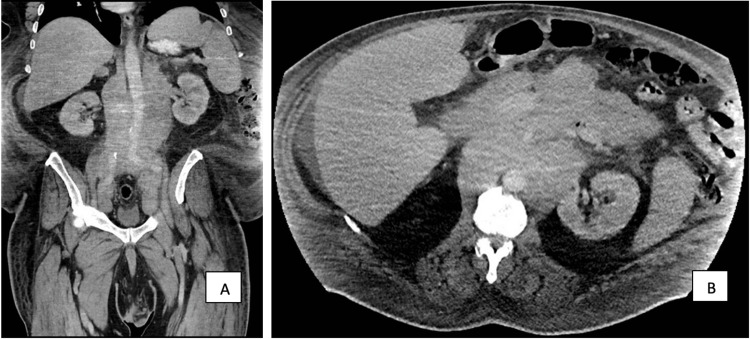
Abdominal CT showing large lymphomatous mass: (A) coronal, (B) axial.
